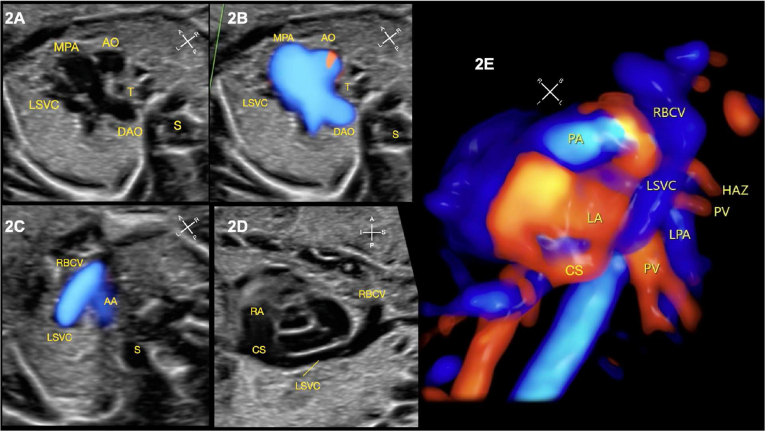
2-and 3-Dimensional Fetal Echocardiography of Isolated Left Superior Vena Cava in a 22 Weeks Fetus (A to E) Isolated LSVC. (A and B) Gray-scale (A) and power Doppler 3-vessel-tracheal (B) views showing abnormally oriented 3 vessels (absent RSVC and persistent LSVC). (C) Upper mediastinal view showing the RBCV. (D and E) Gray-scale (D) and 4-dimensional spatiotemporal image correlation STIC color Doppler (E) images showing the entire LSVC drainage into CS in sagittal view. Note the RBCV draining into LSVC. AA = aortic arch; HAZ = hemiazygos vein; RBCV = right brachiocephalic vein; other abbreviations as in Figure 1.
